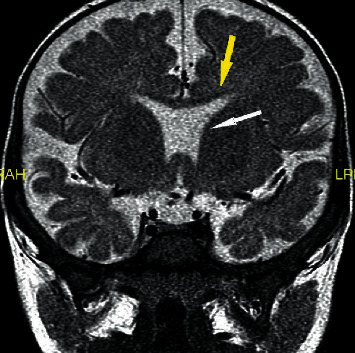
Absence of the septum pellucidum (white arrow) and pointing of frontal horns (yellow arrow) seen on MRI of the brain.
